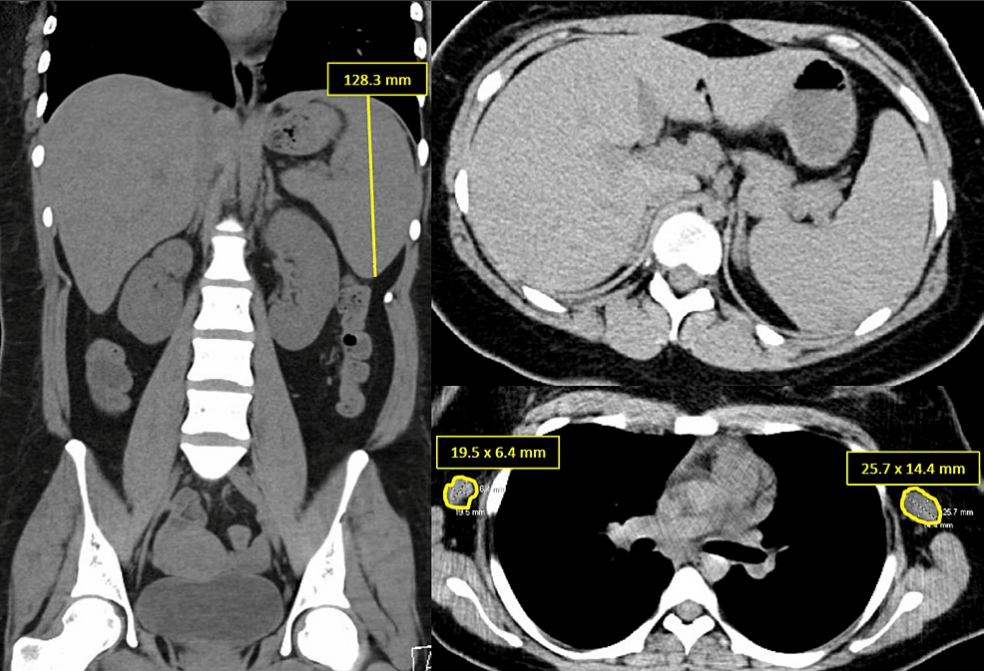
CT of the chest, abdomen, and pelvis revealing splenomegaly and axillary lymphadenopathy
